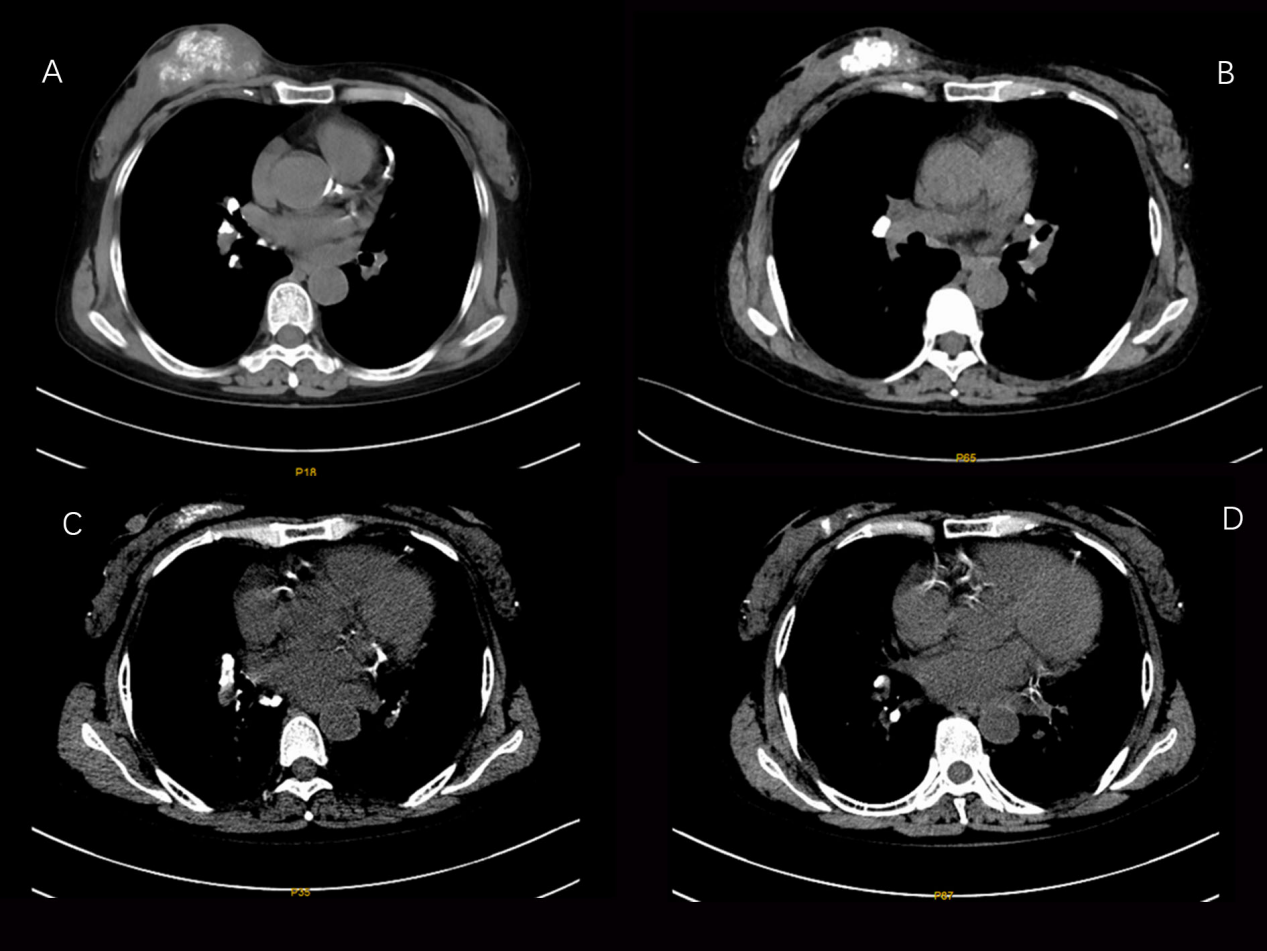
CT images of changes in breast tumor lesions in patients during the treatment period from August 6, 2021 to February 25, 2022 (A:2021-08-06 B:2021-09-25 C:2021-12-17 D: 2021-02-25).
